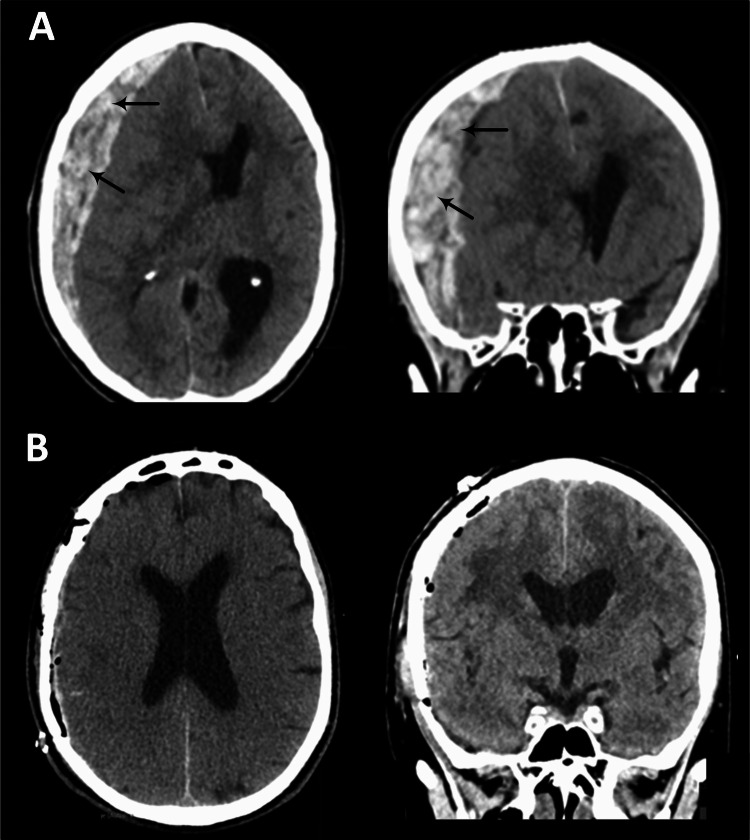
Acute subdural hematoma (aSDH) before and after craniotomy. A non-enhanced computed tomography (CT) scan in a 73-year-old man shows an aSDH (A) before craniotomy (black arrows), and (B) the day after craniotomy with evacuation of the bleed. The gMRI was performed 2.8 months after surgery. Axial (left) and coronal (right) CT scans are shown
